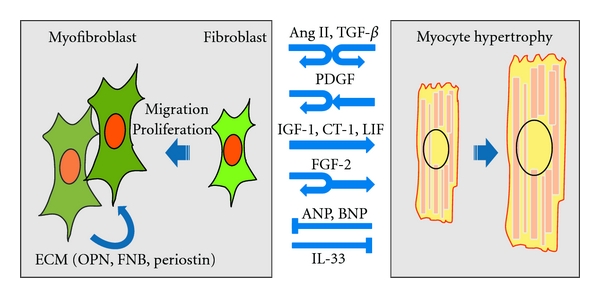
Reciprocal interactions between fibroblasts and cardiomyocytes. Many growth factors and cytokines have been shown to act in autocrine and/or paracrine fashion to induce hypertrophic responses in cardiomyocytes and activate fibroblasts. This scheme depicts only some of the factors identified. Ang II: angiotensin II; TGF-*β*: transforming growth factor-*β*; PDGF: platelet-derived growth factors; IGF-1: insulin-like growth factor-1; CT-1: cardiotrophin-1; LIF: leukemia inhibitory factor; FGF-2: fibroblast growth factor 2; ANP: atrial natriuretic peptide; BNP: brain natriuretic peptide; IL-33: interleukin-33; ECM: extracellular matrix; OPN: osteopontin; FBN: fibronectin.
